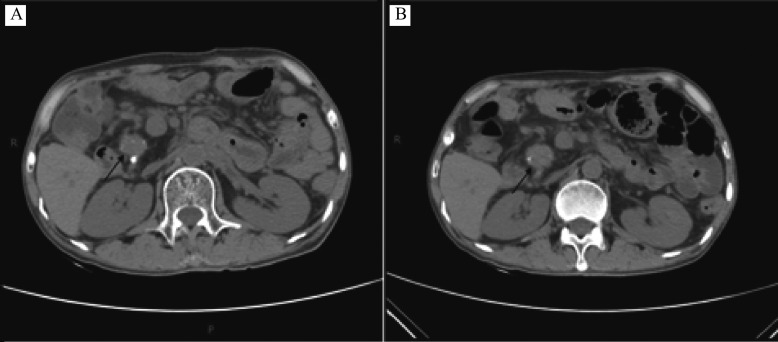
Computed tomography scan images.
